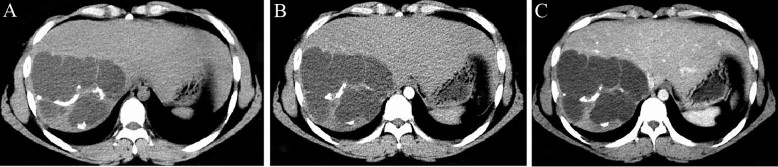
(Patient 3) Abdominal contrast-enhanced CT scan shows a non-enhancing, circular, fluid-density lesion with multiple septations and partial calcifications in the non-contrast phase. The contrast-enhanced scan reveals enhancement confined to the relatively thick septations within the lesion.
